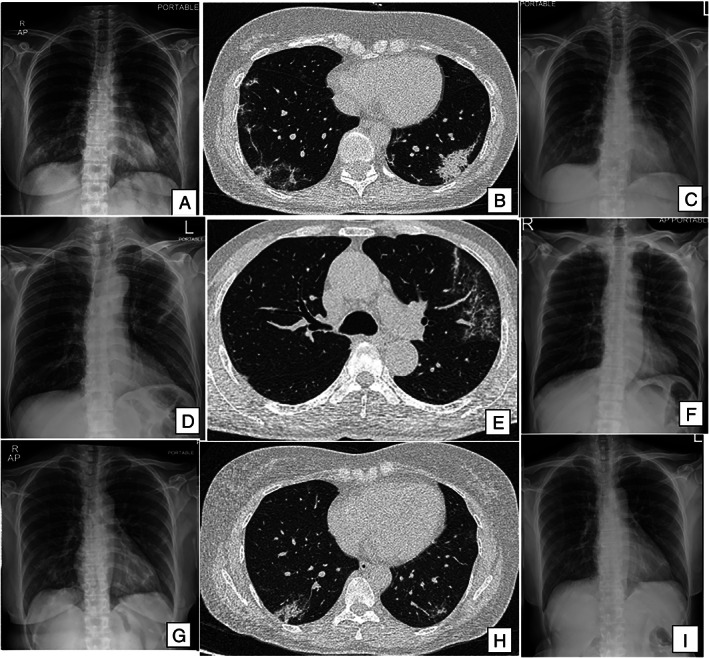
(A, D, G) Chest radiographs on admission showing various degrees of pneumonia. (B, E, H) High‐resolution computed tomography (HRCT) scans showing both ground‐glass opacification (GGO) and consolidation in the three patients. (C, F, I) Repeat chest radiographs showing resolution with treatment.
